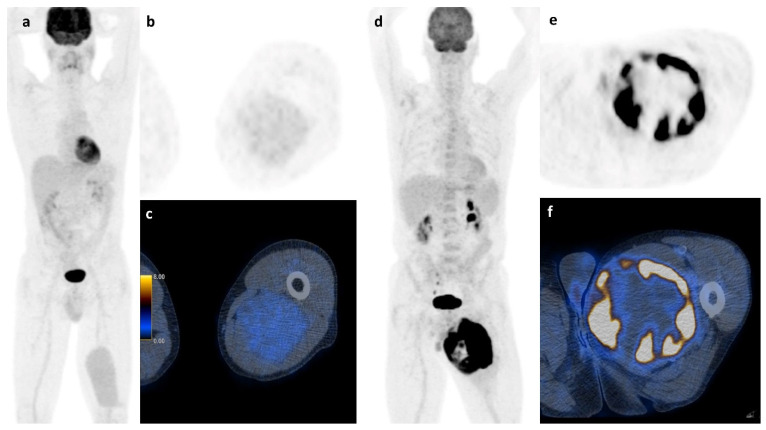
Soft tissue sarcoma: A whole body scan (a) and the axial images (b,c) of a 45 y.o. patient with a myxoid liposarcoma of the left thigh and a survival of at least 107 months shows low FDG-activity (2.35 SUV_max_), while the 73 y.o. patient on the right (d–f) with a pleomorphic undifferentiated sarcoma and high FDG-activity (31.60 SUV_max_) had a survival of only 12 months.
